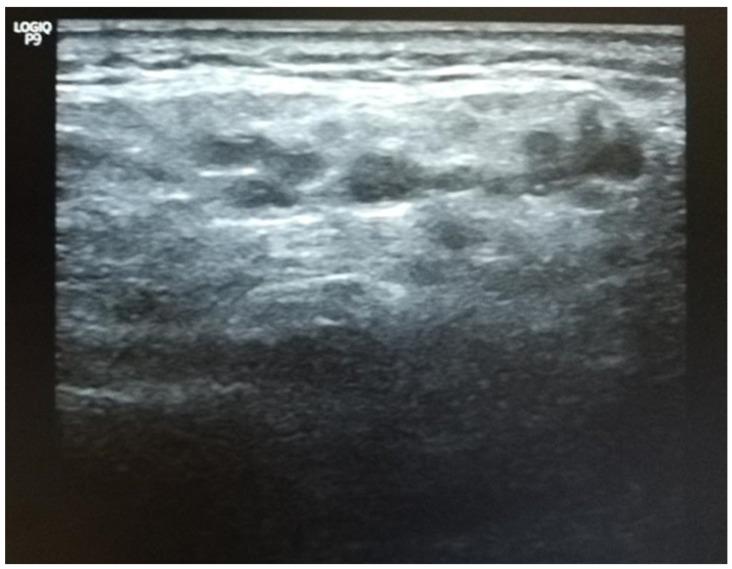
Ultrasound of the patient’s salivary glands. Grade 3, severe changes: diffuse inhomogeneity with anechoic/hypoechoic areas occupying the entire gland surface and surrounded by abnormal tissue and hyperechoic bands, corresponding to the scoring system described by S. Jousse-Joulin et al. [108]. Courtesy of Ciprian Jurcut, Internal Medicine Department, “Dr Carol Davila” Central Emergency University Military Hospital Bucharest, Romania.
